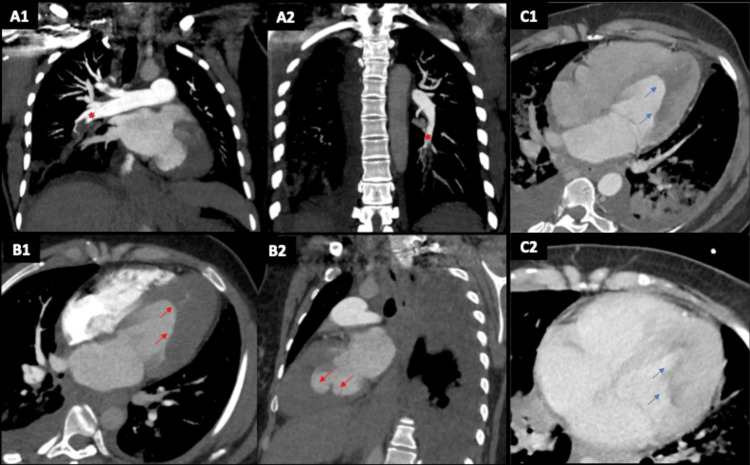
Computed tomography with pulmonary angiography These images reveal multiple bilateral lobar pulmonary embolisms (panels A1-A2, red asterisks) and also obliteration of the left ventricular apex (panels B1-B2, red arrows) by a subendocardial hypodensity structure, highly suspicious of being a thrombus, after early arterial and delayed contrast CT phases (panels C1-C2, blue arrows).
